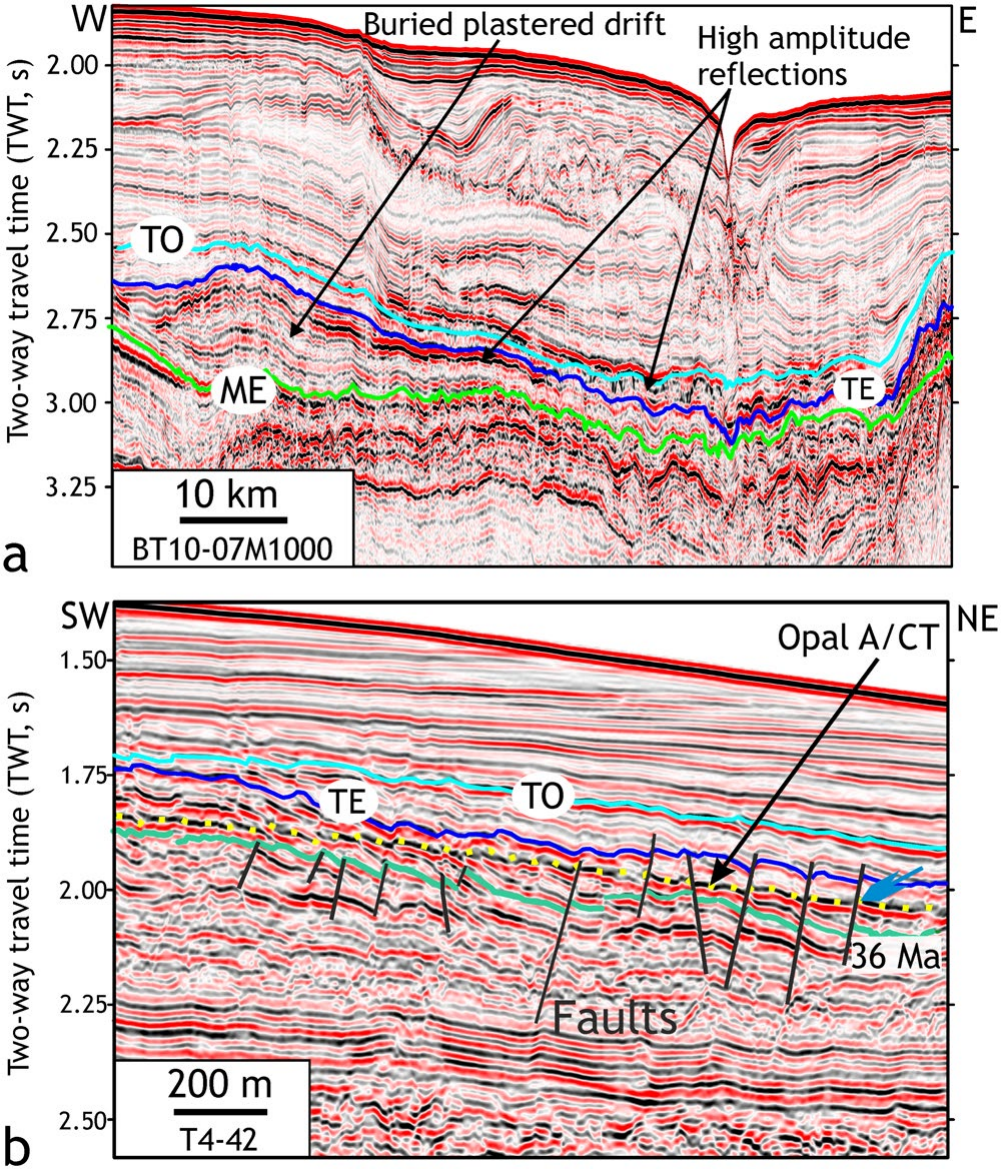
Late Paleogene sedimentary drifts and opal-A/opal-CT reflector. (a) A lenticular shaped unit between markers ME and TE is identified as a buried plastered drift south of the Bounty Trough. Strong positive amplitude reflections are seen towards the top of the drift in the BT. (b) The opal-A/opal-CT reaction front shows negligible or smaller offset (blue arrow) than the offset of the host Late Eocene unit (cf. ref.^75^). The diagenetic transformation could have post-dated the displacement across the faults, or the displacement rate of the faults was greater than the upward advancement of the diagenetic front. Some faults extending into the Oligocene and Miocene sequence also affected the diagenetic front, probably post-dating diagenetic transformation.
